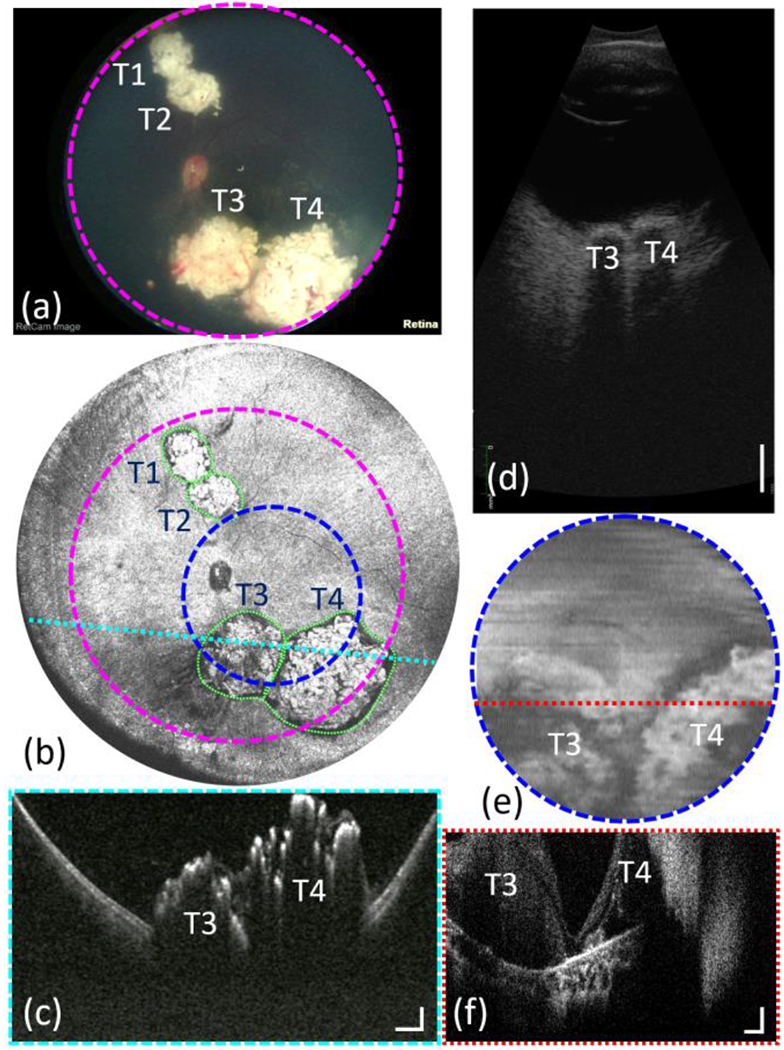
(a) RetCam color fundus image from a patient with multifocal retinoblastoma (tumors labeled as T1-T4). (b) *En face* OCT image obtained by our contact handheld SS-OCT system. (c) Selected B-scan image corresponding to the location of dashed cyan line. Scale bars are 1 mm (horizontally) and 500 μm (vertically). (d) Selected ultrasound B-scan image. Scale bar is 5 mm. (e) *En face* OCT image taken by Leica handheld OCT (Envisu C2300, Leica Microsystems, Germany). The imaging area was marked by dashed blue circle in (b). (f) Selected B-scan image corresponding to the location of dashed red line. Scale bars are 500 μm (horizontally) and 200 μm (vertically).
